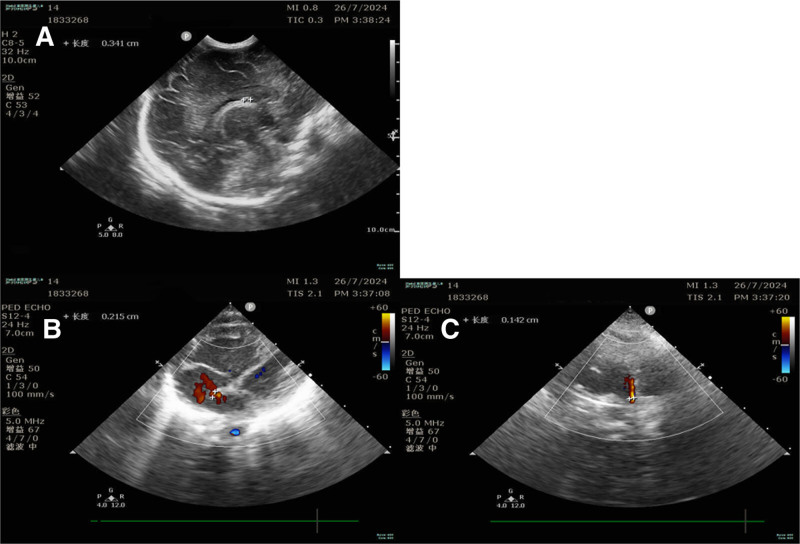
(A) Cranial ultrasound examination revealed subependymal cysts. (B and C) Echocardiography showed a patent foramen ovale (PFO) with a diameter of 0.21 cm. and a patent ductus arteriosus (PDA) with a diameter of 0.14 cm (C).
